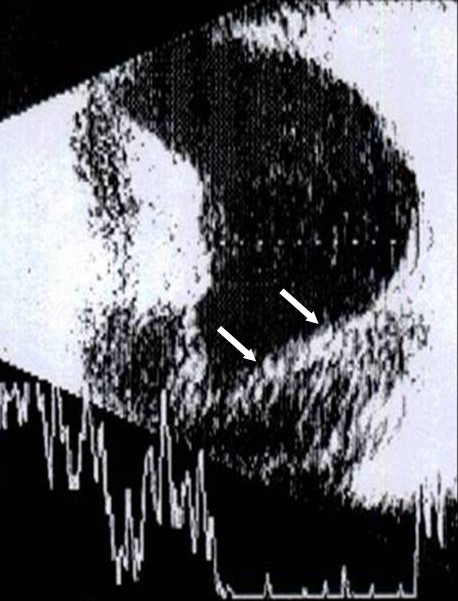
Ultrasonography (right eye) at presentation, indicating low retinal detachment and vitreous hemorrhage.
